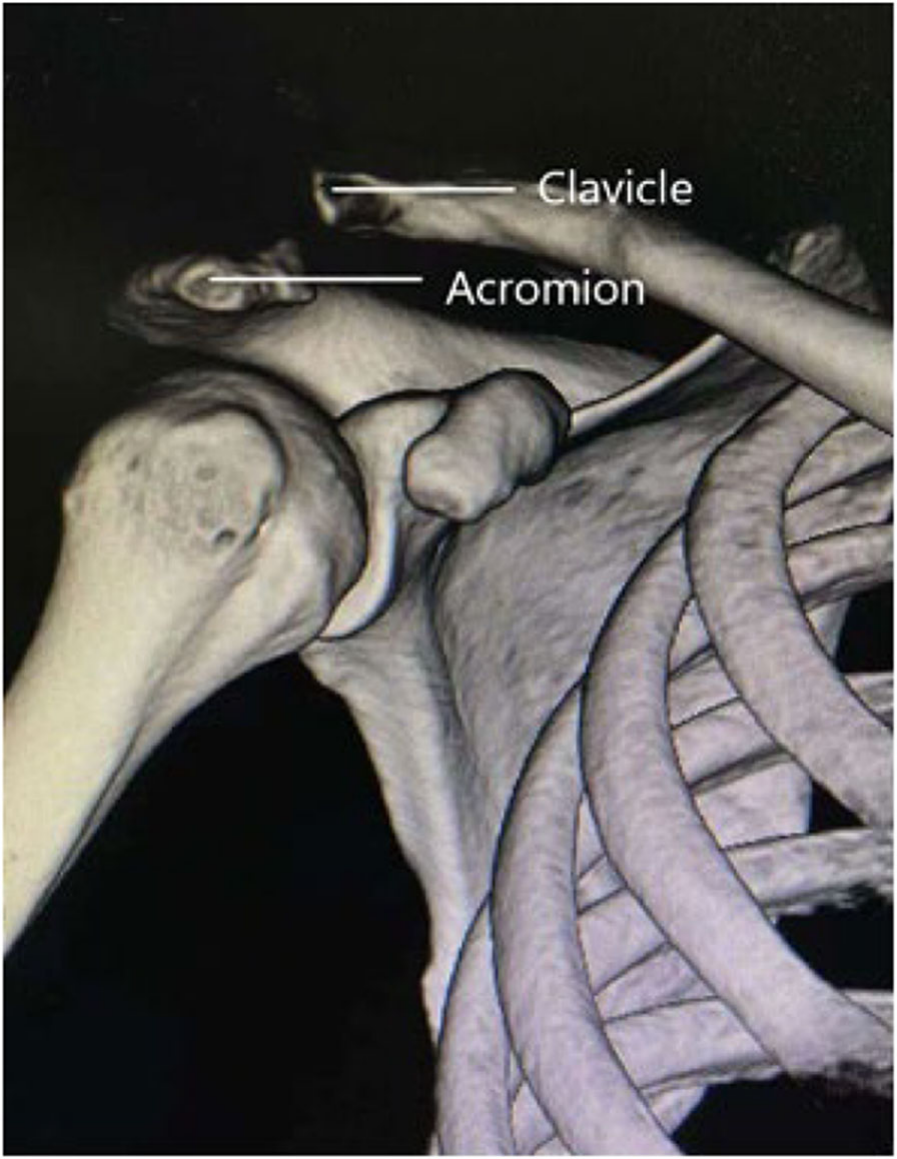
Preoperative CT image of a 32-year-old man who fell and sustained a right acromioclavicular joint dislocation
